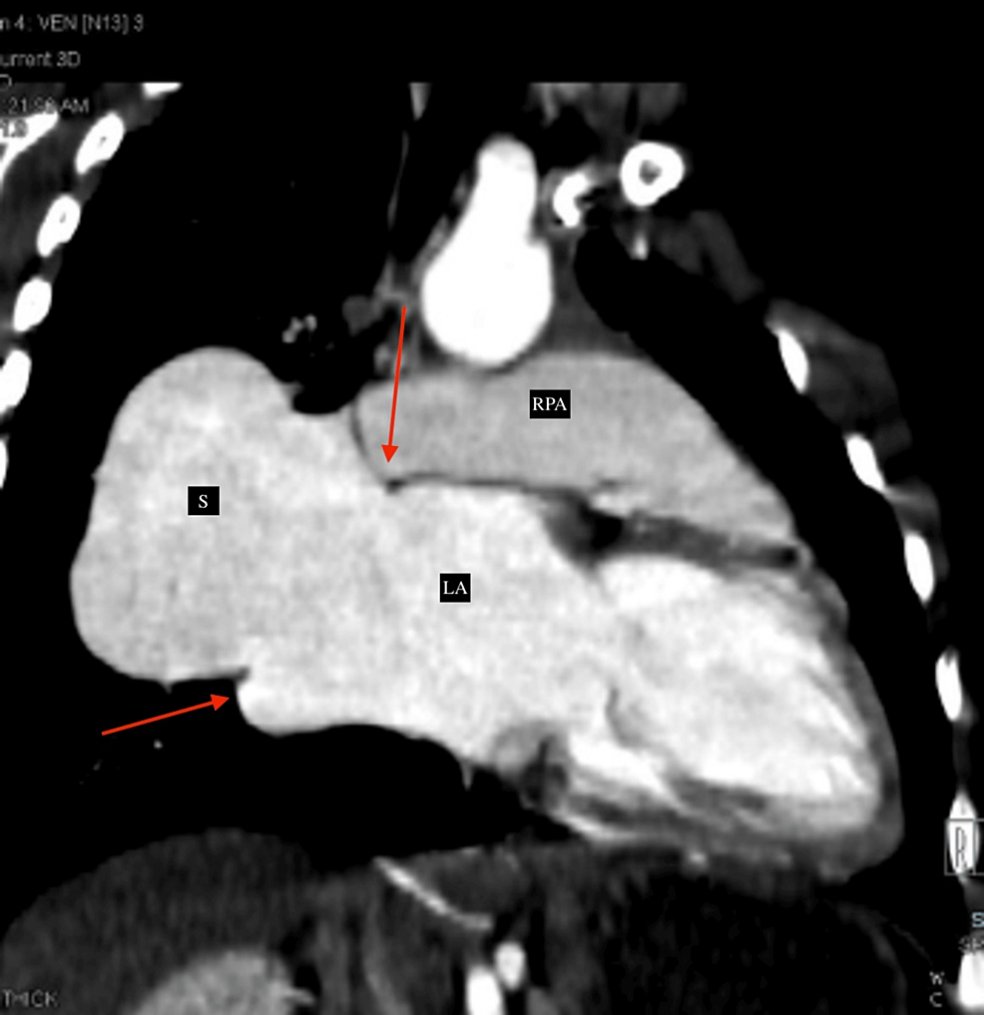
CT reformatted image (coronal section) demonstrating the dilated aneurysmal sac (labeled as "S") draining into the left atrium (labeled as "LA"). The site of communication between the sac and the left atrium is marked by red arrows). RPA: right pulmonary artery
